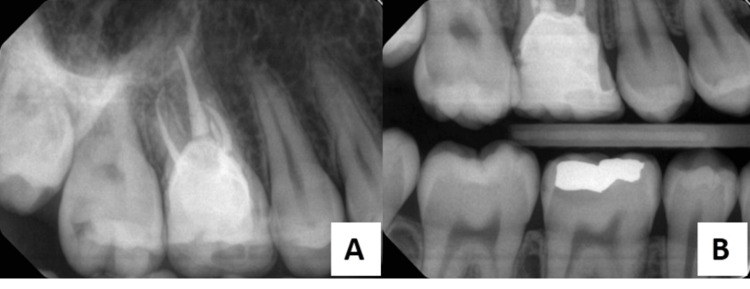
(A) Two-year follow-up periapical radiograph; (B) Two-year follow-up bitewing radiograph.
